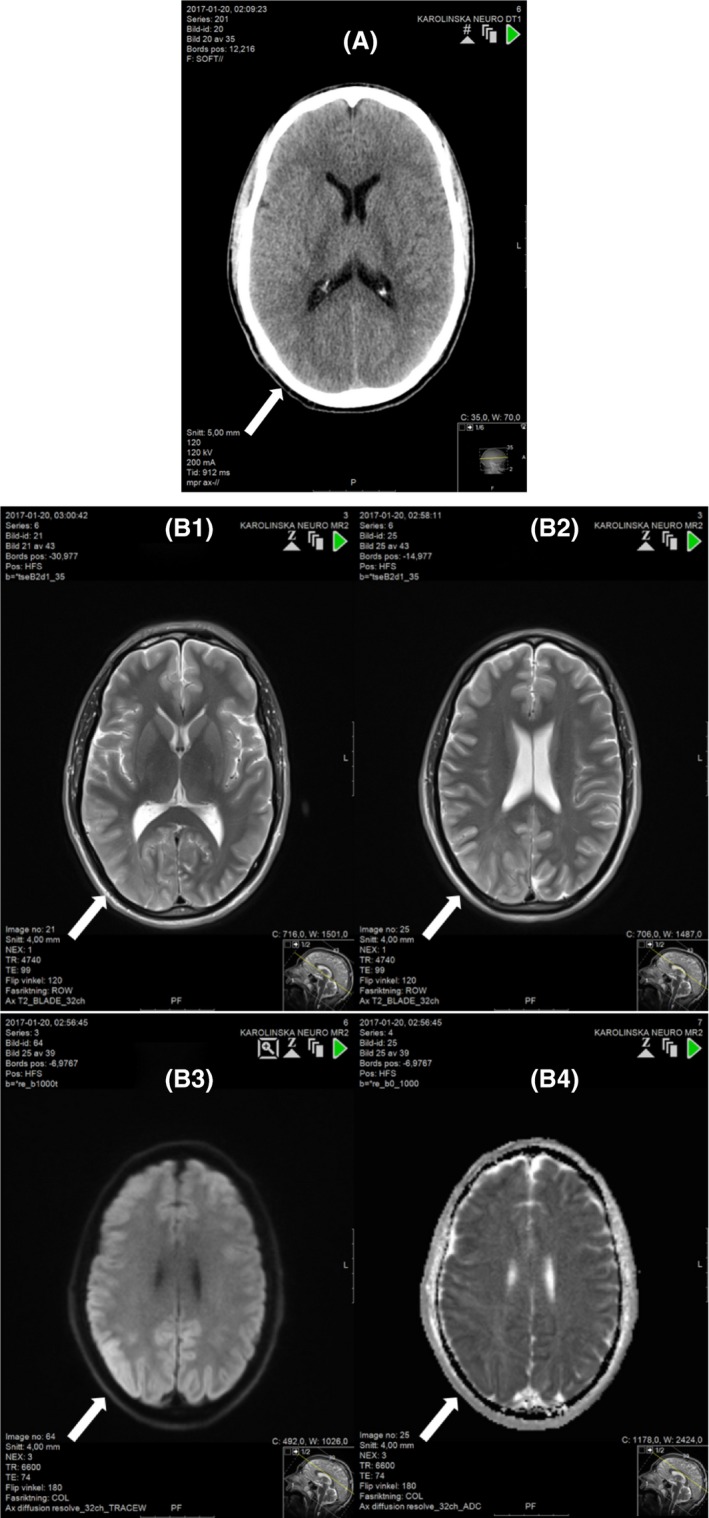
A computed tomography CT scan 12 hours after initial CT showing normalisation of attenuation and only slight swelling in the right parietal area. (A). T2 weighted images in MRI is almost normal 14 hours after initial CT, only diffuse high signal in cortical areas and white matter in the posterior right hemisphere (1‐2). Diffusion weighted images showed restricted diffusion in large cortical areas, on the right side. The restricted diffusion was interpreted as ischemic areas, but with the important notion that the mechanism of air embolism is not the same as vessel occlusion (3‐4) (B).
